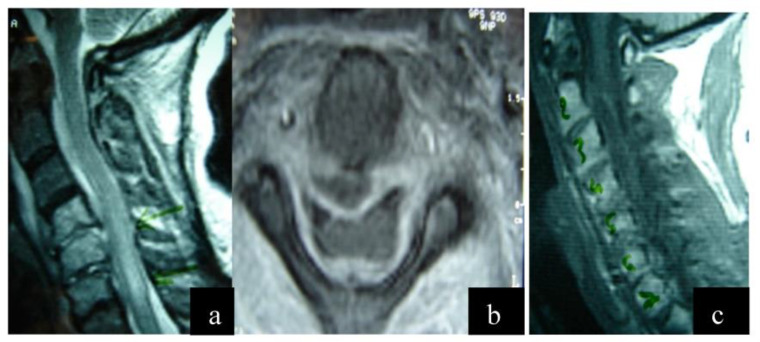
(a) Preoperative magnetic resonance imaging (MRI) sequence T2 lateral view. There is a cervical epidural abscess within the spinal canal below the posterior longitudinal ligament extending from C1 to C5, deformation of the signal of the spinal cord due to an inflammatory reaction. (b) Preoperative magnetic resonance imaging (MRI) sequence T2 axial view. The presence of a pathological cavity below the posterior longitudinal ligament is observed, causing compression of the thecal sac. (c) Preoperative magnetic resonance imaging (MRI) sequence T1 lateral view.
